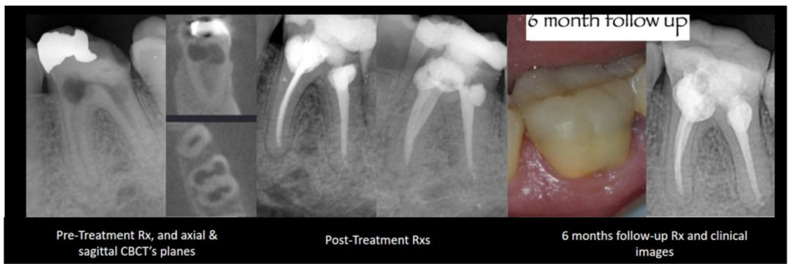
Mandibular first molar with two internal root resorption lesions without perforation that was obturated with GP and ERS. X-ray images of pre- and post-treatments and from a 6-month follow up.
